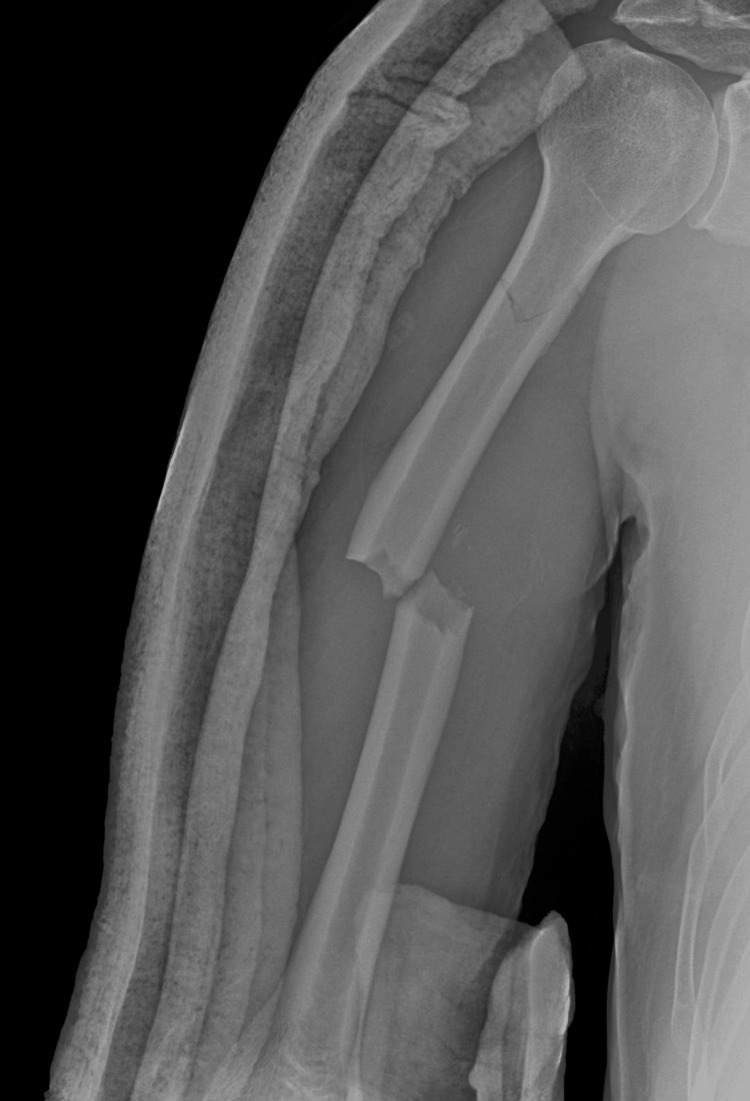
Plain radiograph shows right humerus fracture on the day of the accident
